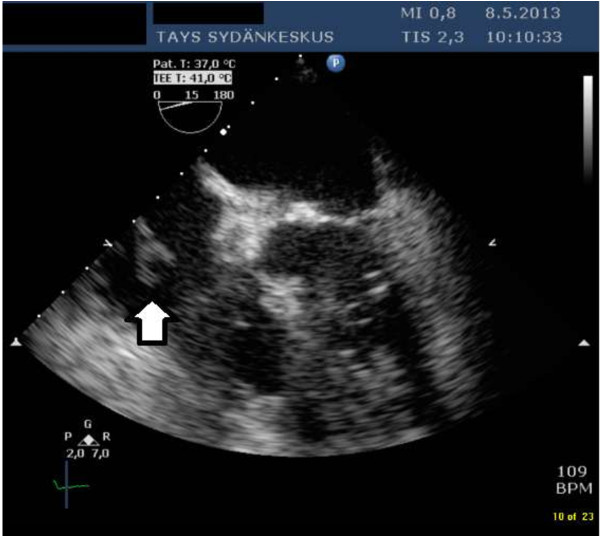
Transesophageal echocardiography examination of the first patient. The bold arrow points to the two-tailed vegetation in the right atrium. The vegetation was attached to the pacemaker lead.
